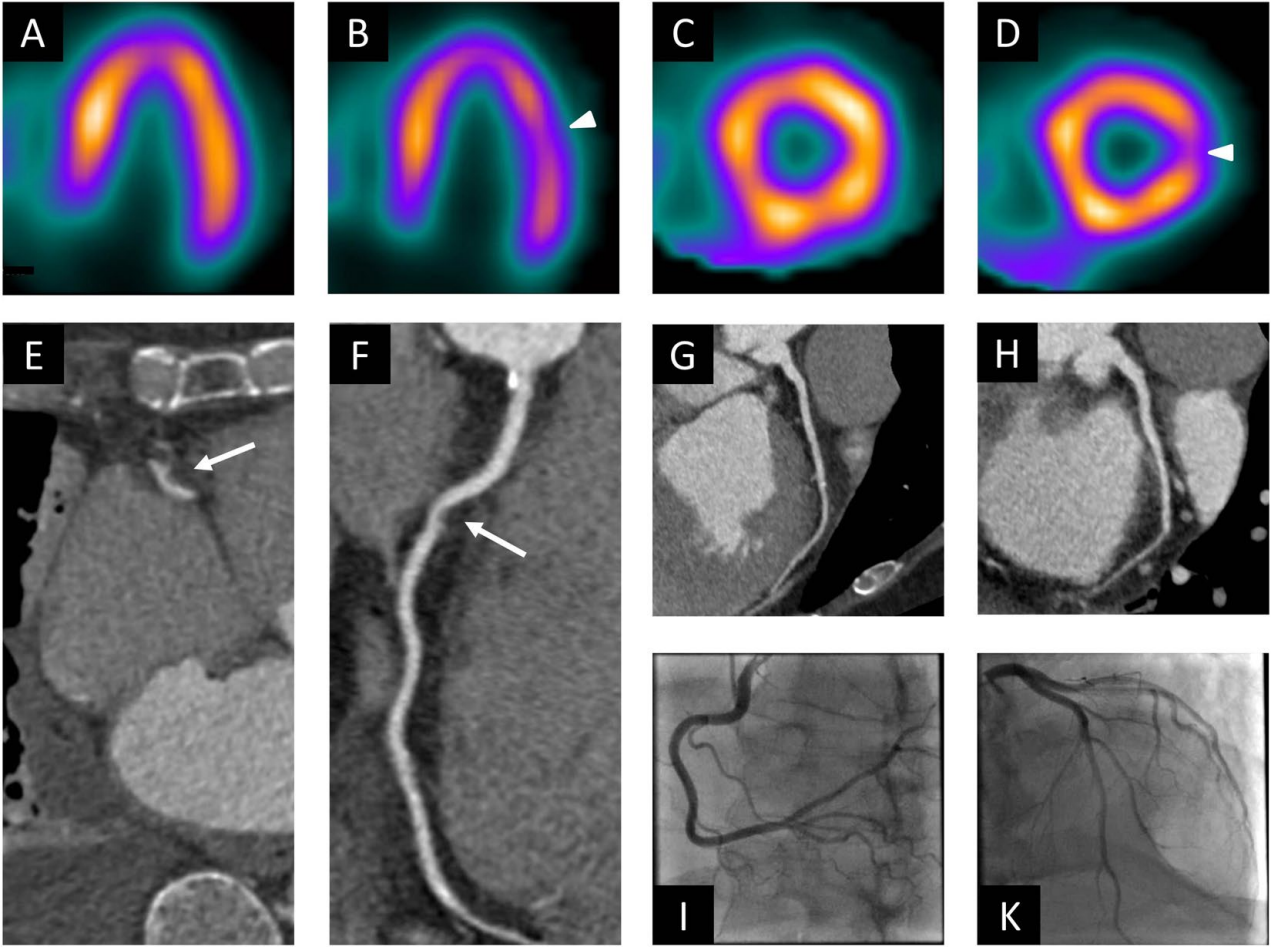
Example of a false positive CCTA examination. This 63 years old male patient underwent stress nuclear myocardial perfusion imaging because of exertional dyspnea. Stress images in the vertical short axis (B) and horizontal short axis views (D) are suggestive of decreased perfusion to the lateral wall of the left ventricle (arrow heads), which is reversible at rest (A,C). The examination was thus interpreted as concerning for lateral wall ischemia. At CCTA, transverse section (E) and curved multiplanar reformat (F) of the right coronary artery were interpreted as showing 50% stenosis with focal soft plaque (arrow). No luminal narrowing is noted in the left anterior descending (G) and circumflex (H) arteries. On catheter angiograms (I,K), there is no evidence of luminal narrowing in any vessel. The false positive finding on CCTA likely represents an artifact. CCTA = coronary computed tomography angiography.
